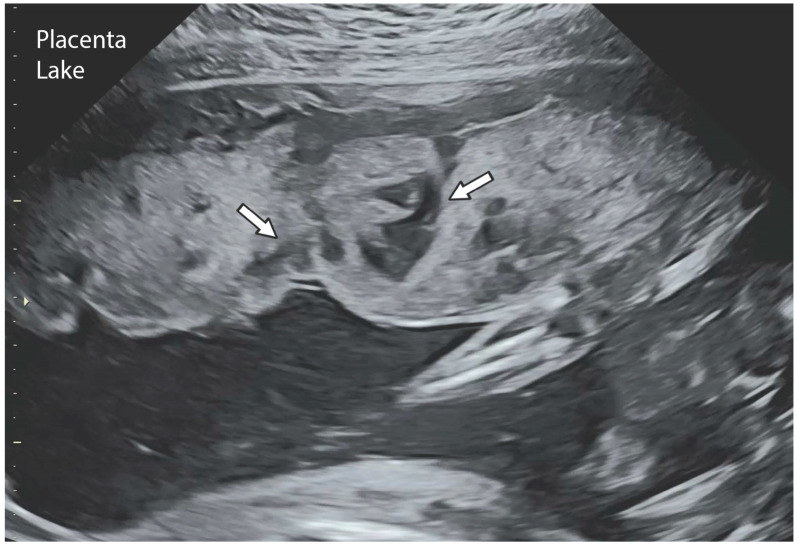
Placental lake on ultrasound. The arrows indicate placental lakes on ultrasound.
